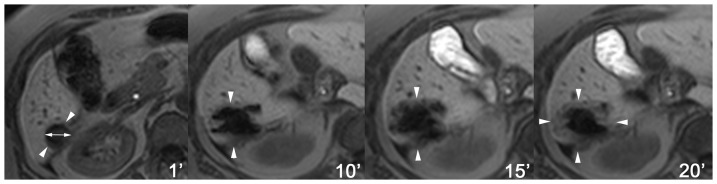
MR thermometry at late phase contrast-enhanced imaging. Thermometric MR imaging (T1 FLASH 2D) delineates the development of a T1-hypointense zone of thermal impact (arrowheads show boundaries) encountering a 2 cm hepatic metastasis (double-headed arrow). Notice that metallic applicator mandrins have been replaced by non-visible laser fibers.
